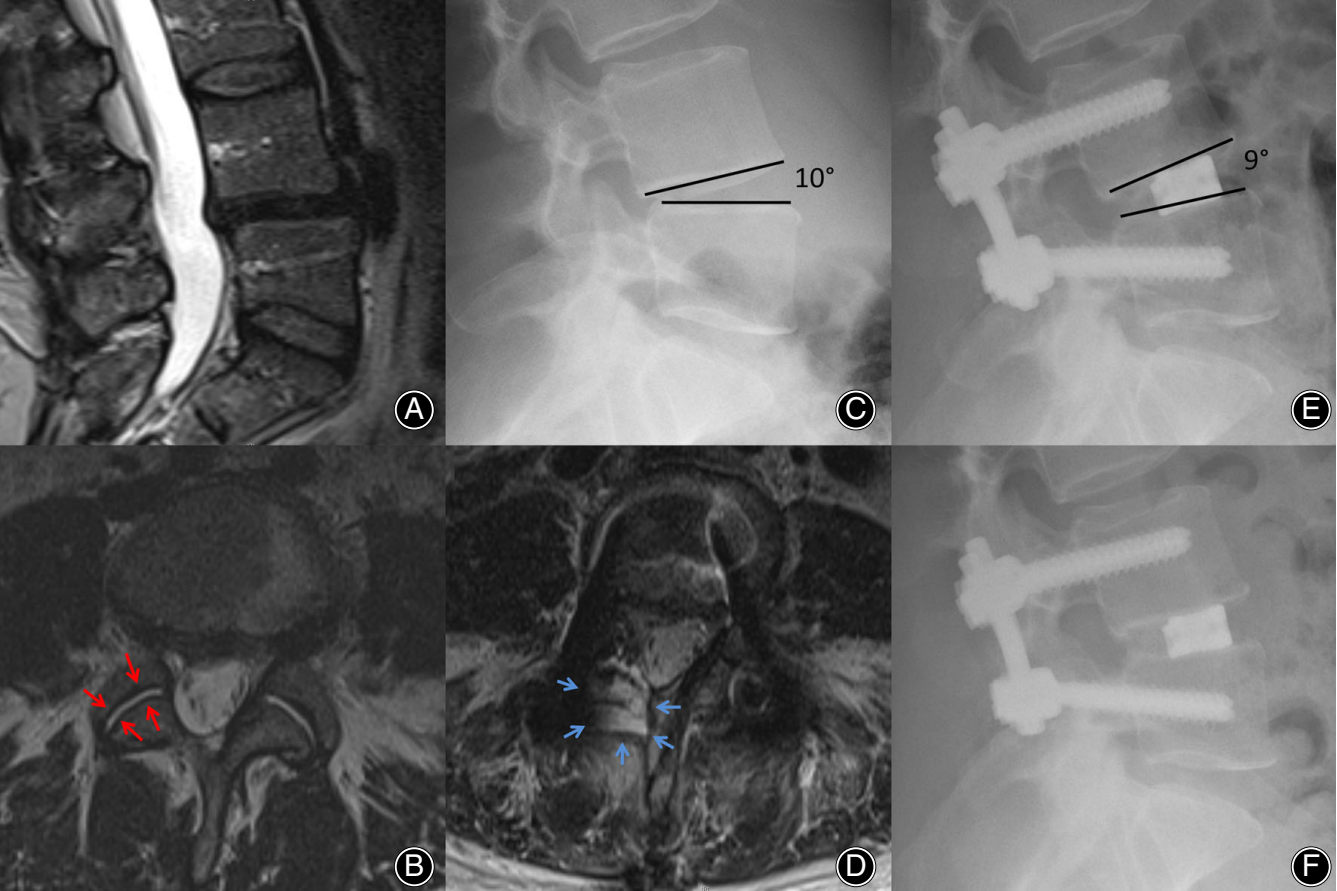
(A) Preoperative MRI scan of a 70‐year old man with an instability of L4/5 after previous decompression surgery. (B) Transverse view (MRI) at level L 4/5 with fluid sign (red arrows) as a sign of instability (C) The preoperative radiograph in a standing position shows segmental hyperlordosis of 10° and a retrolisthesis. (D) Three days after surgery the transverse view (MRI) demonstrates an accumulation of liquor after suturing of the dura (blue arrows). (E) The lateral X‐ray shows a slight decrease in segmental lordosis by 1° to 9°. (F) In the follow‐up after 2 years, a secure bony fusion is evident.
